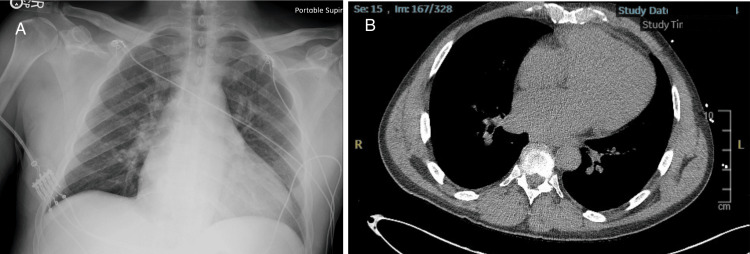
Initial chest radiography (A) and computed tomography of the chest without contrast (B) indicating mild cardiomegaly with no acute findings.
